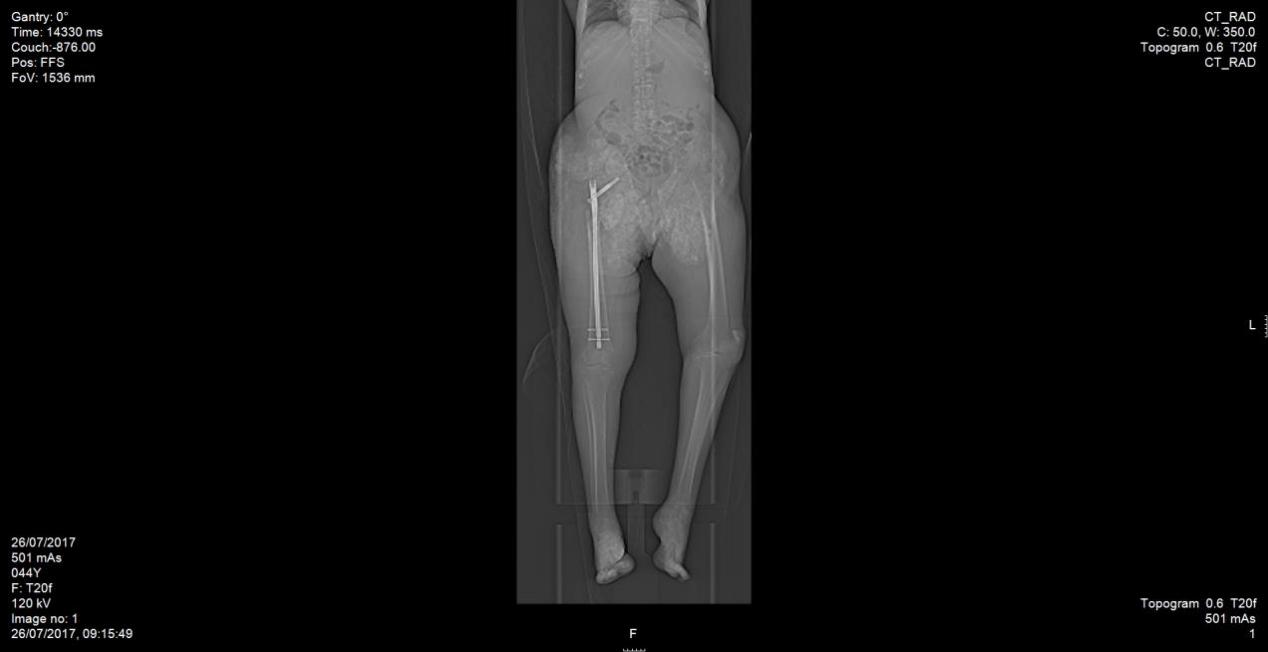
Post-operative CT Scan. During the operation, periarticular calcium deposits made the visualization under X-ray fluoroscopy impossible. Therefore, debulking, open reduction, and internal fixation were mandatory.
